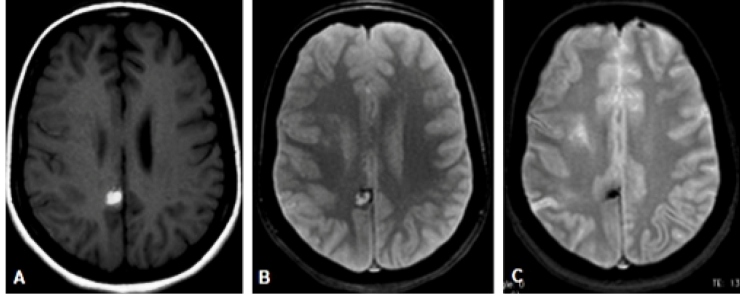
MRI. T1-weighted axial images (A) and T2*-weighted axial images (B and C). A posterior rounded lesion in in the cingulate gyrus, with spontaneous hypersignal on T1 (A) and hyper with hypointense halo on T2* (B), related to parenchymal hematoma. Control examination shows hematoma in the chronic stage, with reduction in size and marked hypointensity on T2* related to hemosiderin (C). MRI: Magnetic resonance imaging; FLAIR: fluid-attenuated inversion recovery
